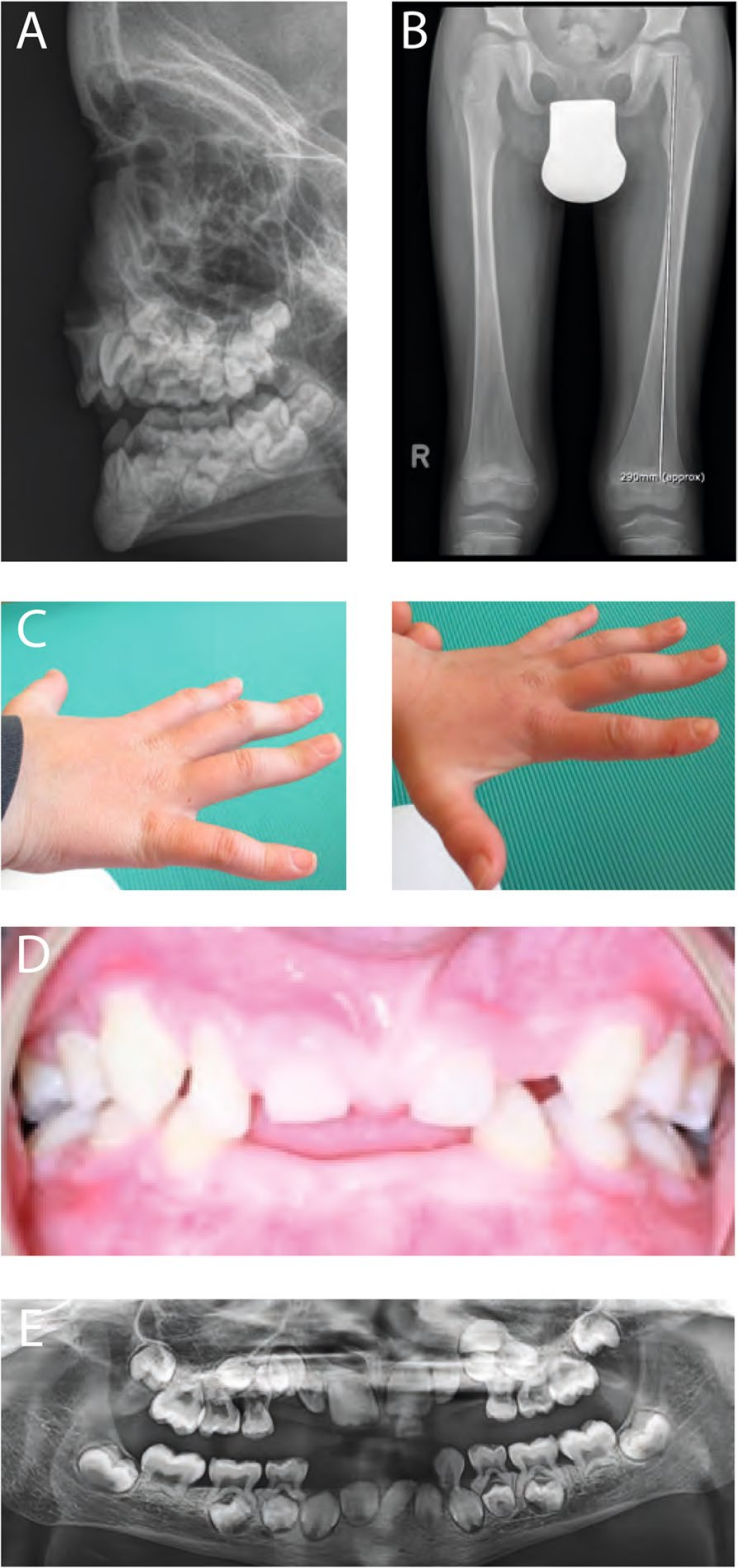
Clinical findings. A Lateral X-ray image of facial bones showing absence of nasal bone. B Anterior–posterior X-ray of the hip and femurs showing hypoplastic acetabular roofs, marked osteopenia of femoral bones and enlarged CCD angles. C Boutonniere deformity of hands. D Image of the teeth at the age of nine years, showing defective dentition with hypoplastic teeth. E Anterior–posterior x-ray of the denture showing aplasia of teeth no. 18, 28, 31, 38 and 48, as well as hypoplastic shortened roots of most of remaining teeth (courtesy of Dr. Zettel)
